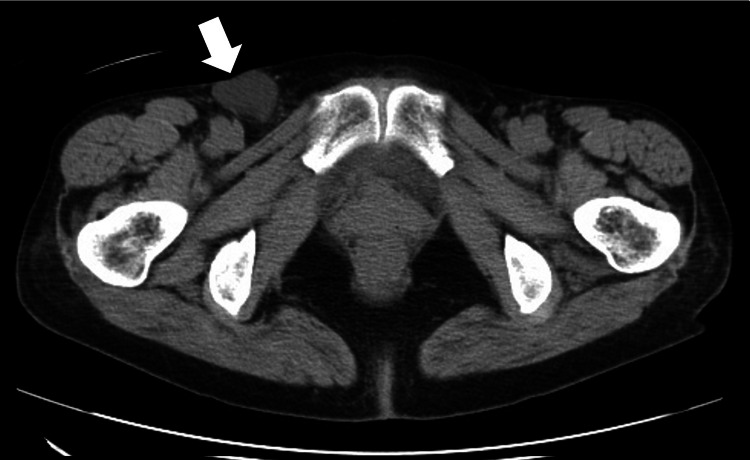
Preoperative plain abdominal CT scan Plain abdominal CT scan showing a suspicious lesion in the right hydrocele of the canal of Nuck (arrow) CT, computed tomography
